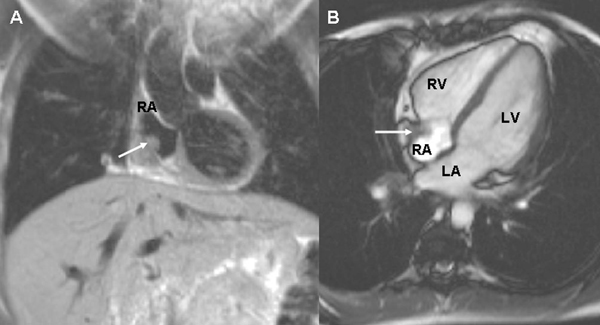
Cardiac magnetic resonance imaging showing the position of the thrombus (arrow) in coronal view (A) and transverse view (B). RV = right ventricle, LV = left ventricle, LA = left atrium and RA = right atrium. Courtesy of Dr Raad Mohiaddin.
